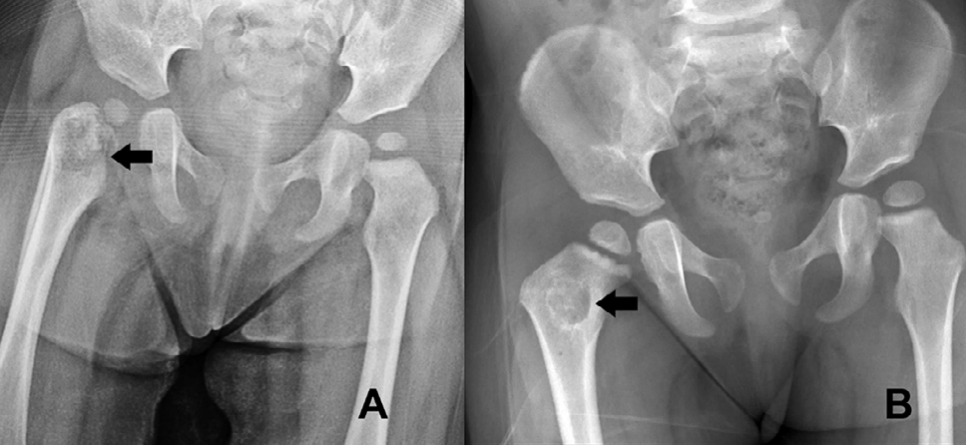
Radiographs of the hips of patient 2 are shown before surgery (A) and at 12-month follow-up visit (B). The arrows represent an osteolytic lesion at the right proximal femur.
